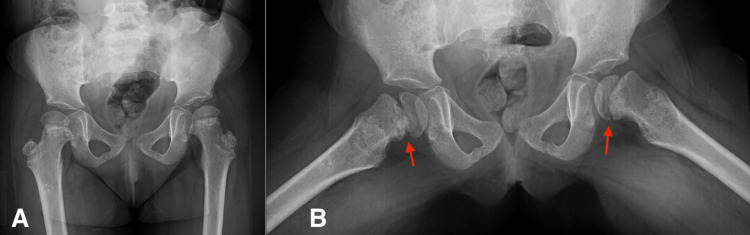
AP pelvis (A) and frog legs lateral (B) X-ray. Redemonstration of bilateral physeal widening, proximal femoral metaphyseal, and iliac crests irregularities, most likely related to chronic kidney disease. On Figure 3B, there is bilateral posterior medial slippage of the femoral epiphysis (red arrows), the right worse than the left, finding are suggestive of bilateral SCFE, with no fractures or gross soft tissue abnormalities. SCFE, slipped capital femoral epiphyses
